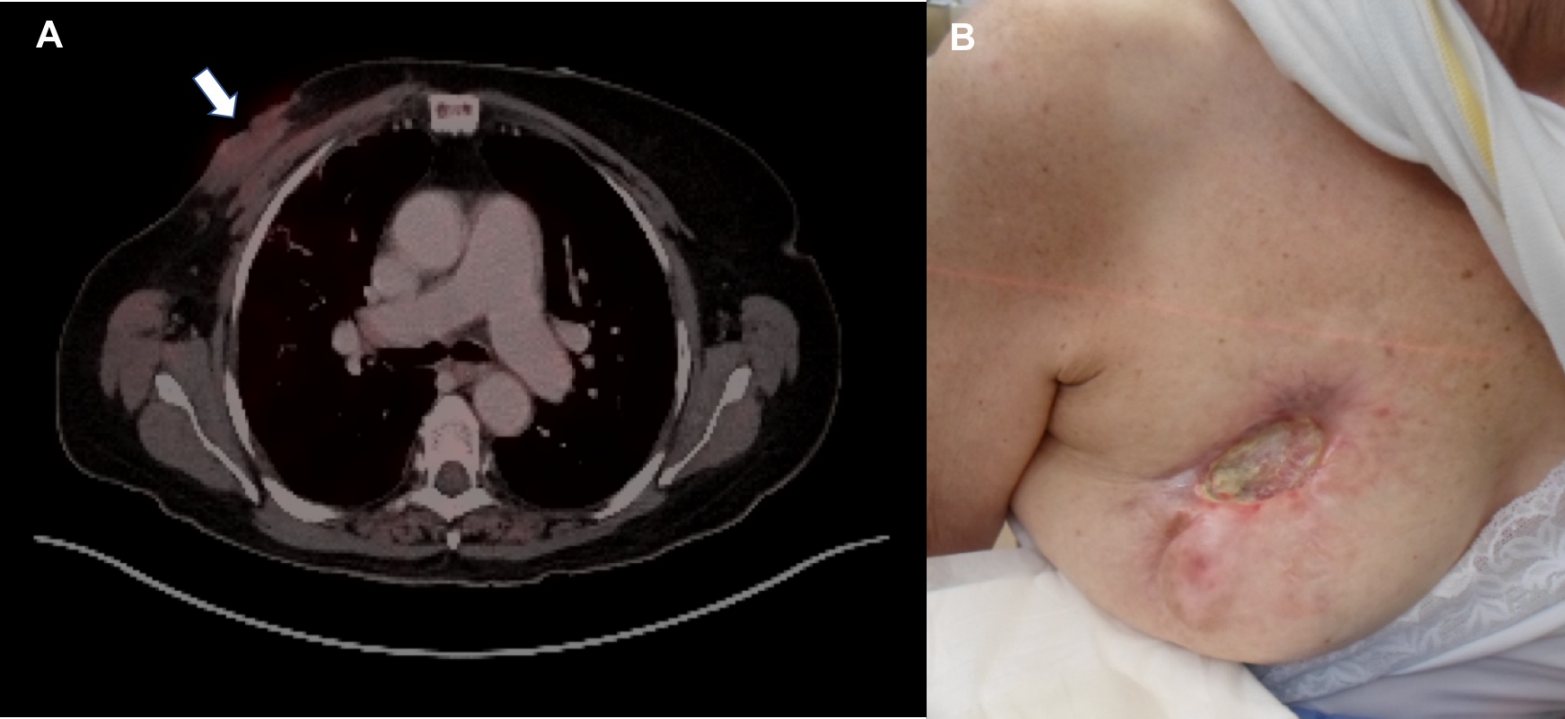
Response of the Breast Mass to Radiation Therapy (A) Fluorodeoxyglucose positron emission tomography/computed tomography (FDG-PET/CT) scan at four months after completion of radiotherapy (white arrow); (B) Regression of the mass and near closure of the open wound at four months post-treatment on physical exam.
